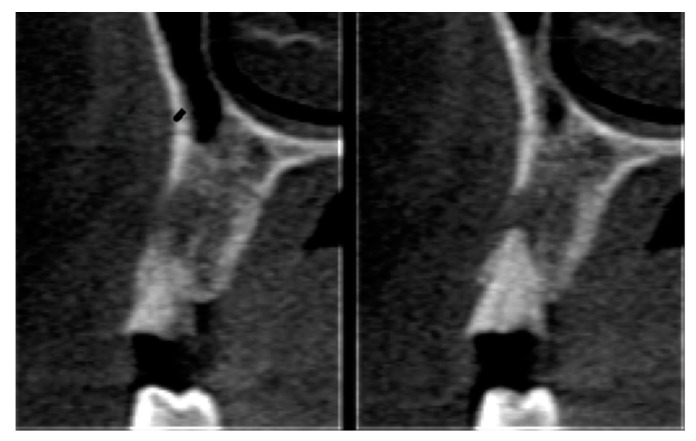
CT images of tooth 14 prior to the procedure.
